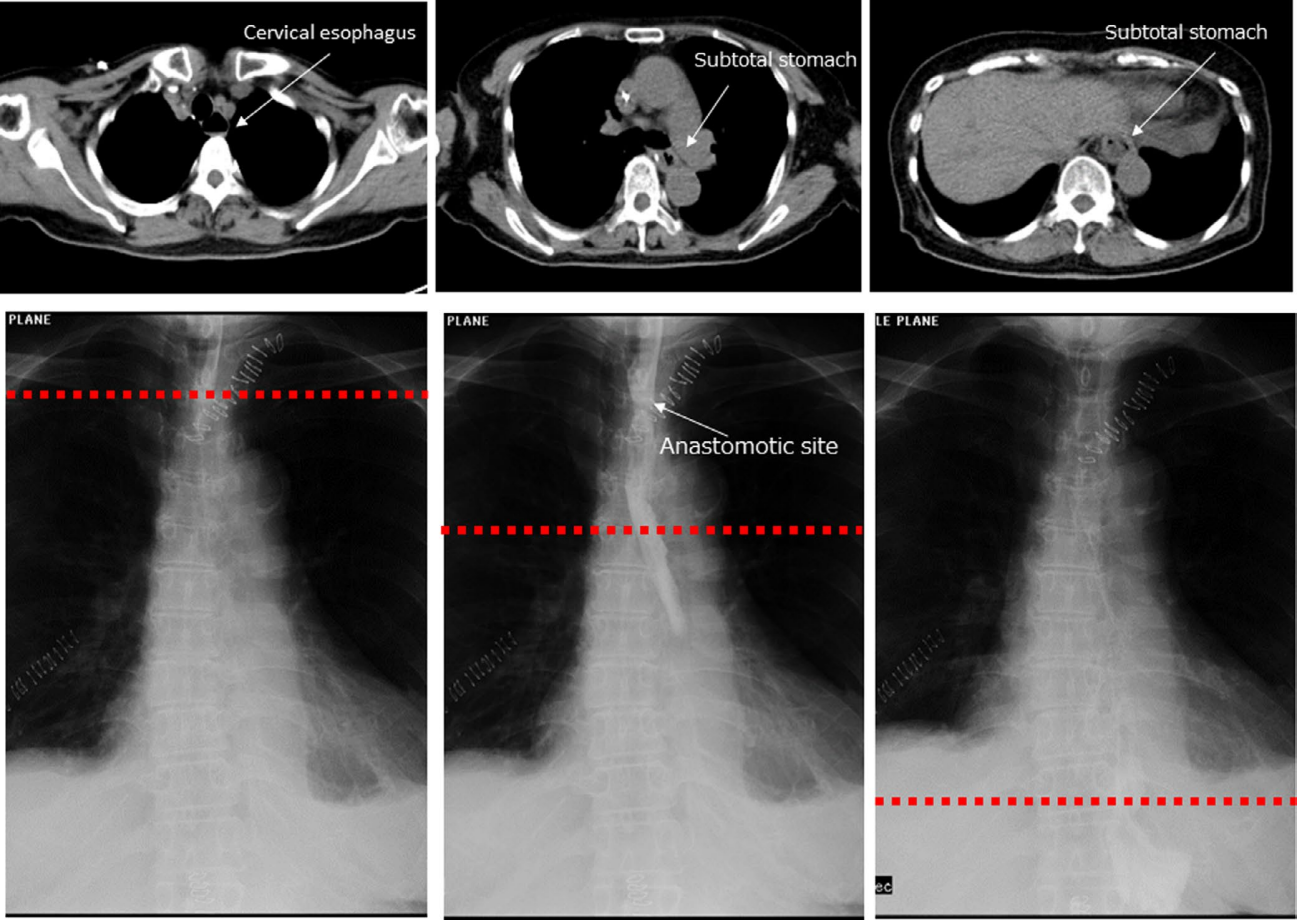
Swallowing test using gastrografin after reconstruction along with contrast‐enhanced computed tomography. Red dotted line indicates the height of the computed tomography slice. The reconstructed stomach is seen located in the original esophageal position without bulging, and gastrografin flows straight down
